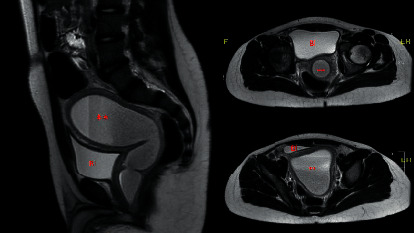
Our patient's MRI of the abdomen and pelvis (sagittal and axial) more clearly demonstrates that the fluid-filled structure located in the midline and posterior to the bladder (Bl) is actually an enlarged prostatic utricle (^*∗∗*^) filled with debris.
